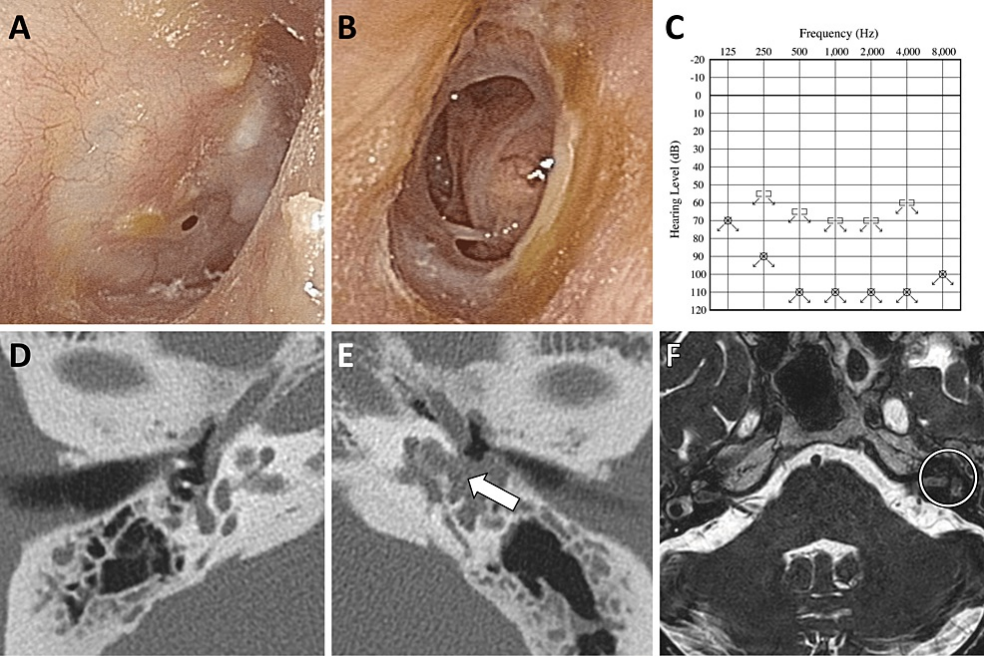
Preoperative clinical findings. (A) Endoscopic finding of the right tympanic membrane. (B) Endoscopic finding of the left tympanic membrane. (C) Pure tone audiogram. (D and E) Axial CT images of the right and left temporal bones, showing soft tissue density with osteolysis of the left cochlea (white arrow). (F) Heavy T2-weighted MR image showing reduced signal intensity in the left inner ear (white circle).
